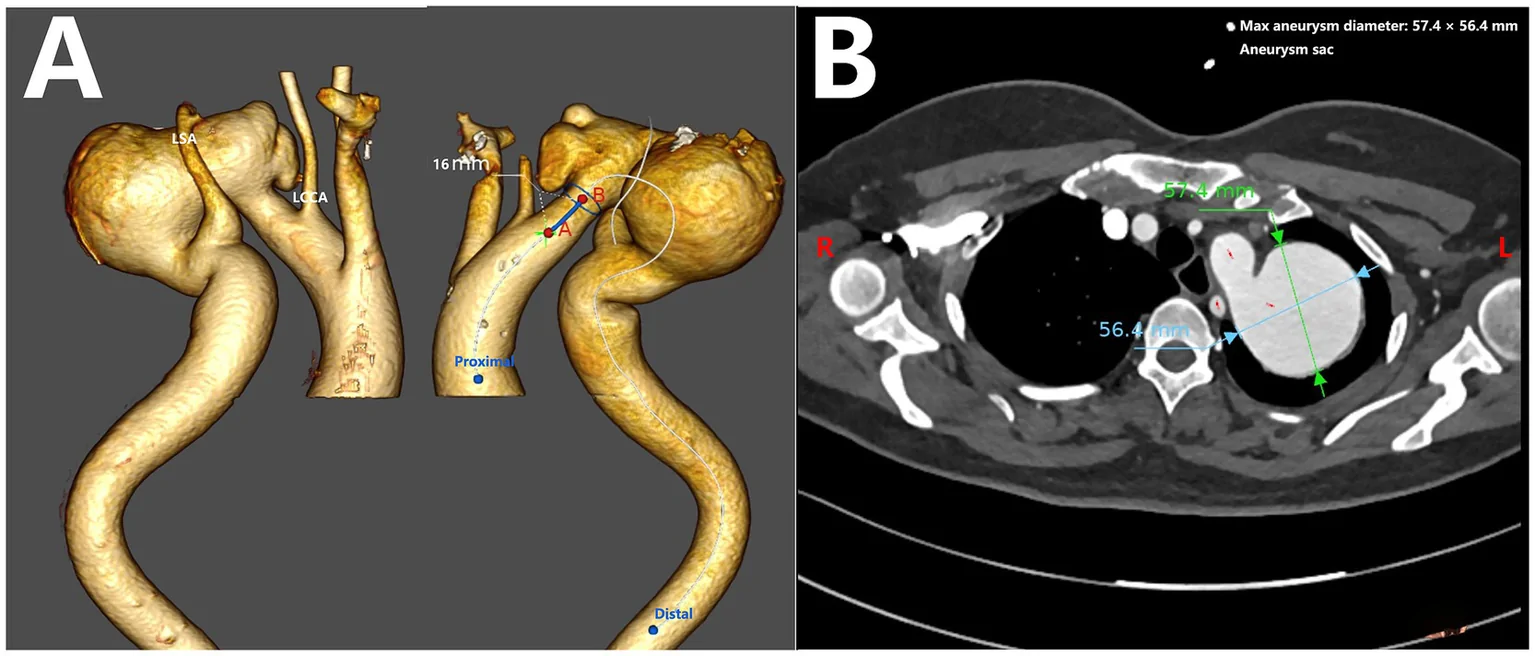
Preoperative CTA showing severe distal arch tortuosity, the reported short proximal landing segment, and aneurysm size. (A) Three-dimensional reconstruction and planning view. The reported 16-mm distance extends from the distal edge of the LCCA ostium to the proximal margin of the aneurysmal segment, measured along the aortic centreline. (B) Axial CTA showing maximal outer aortic dimensions of 57.4 × 56.4 mm. CTA, computed tomography angiography; LCCA, left common carotid artery; LSA, left subclavian artery.
